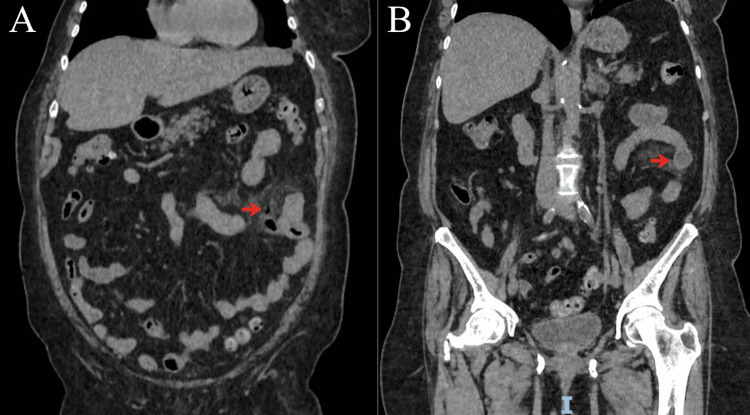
Contrast-enhanced abdominal CT, coronal reformations. A) Perforated jejunal diverticulum on the mesenteric border with peridiverticular fat stranding and a tiny focus of localized extraluminal air adjacent to the sac (arrow), consistent with contained perforation. B) Non-perforated proximal jejunal diverticulum with mural thickening (arrow) and no extraluminal air or upstream obstruction.
